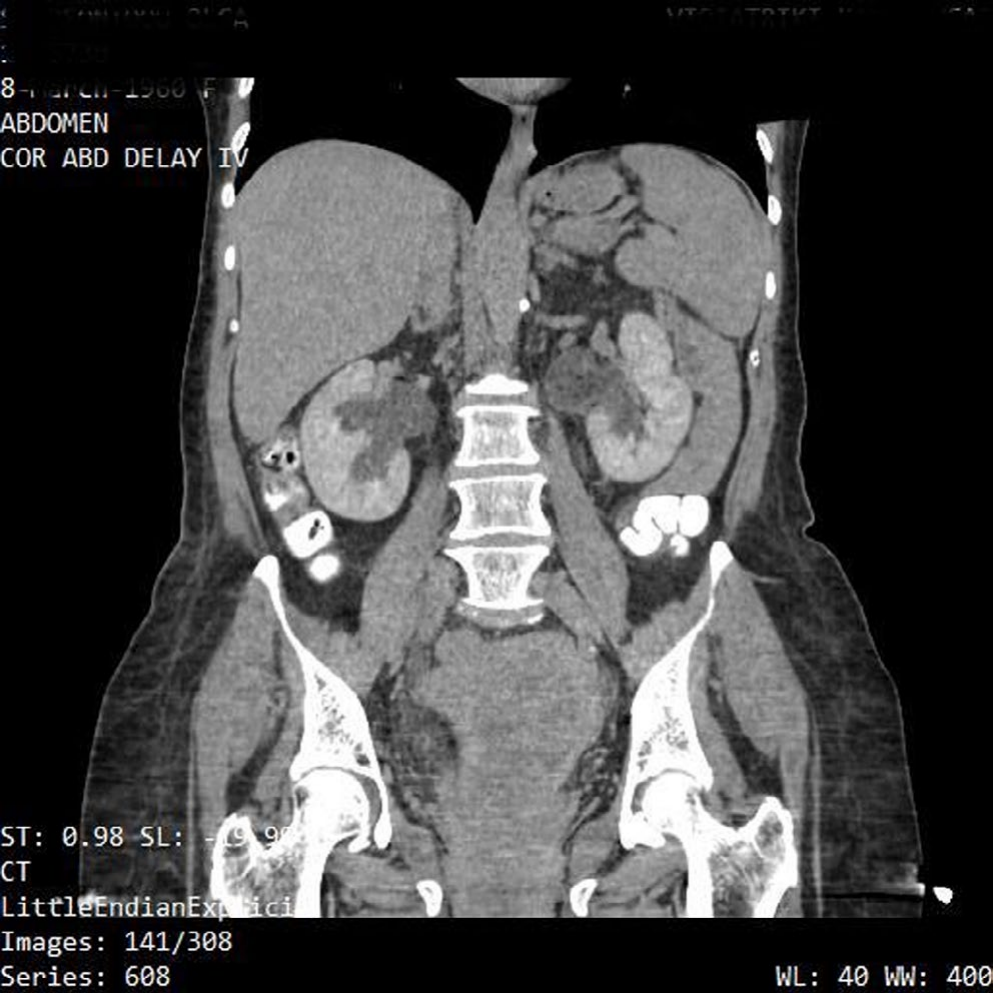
Abdominal CT image in delayed post contrast venous phase, depicting a mildly hypo‐dense lesion with indistinct boarders, and extending from the cervix to the endometrium and the vagina. Mild bilateral hydronephrosis of the kidneys is also noted
